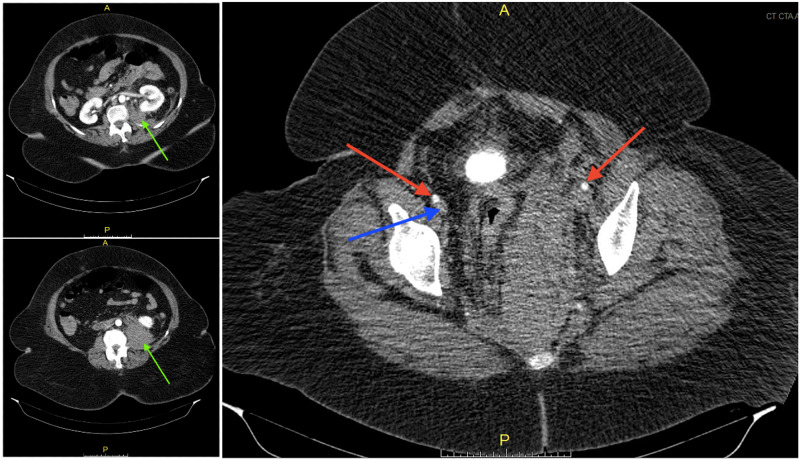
CT angiogram abdominal aorta and bilateral iliofemoral lower extremity runoff with contrast showing a large hematoma (green arrows) extending from the pelvis to the iliopsoas musculature measuring approximately 25 x 10 x 6 cm at the level of the pelvis without extravasation of contrast. The arterial vasculature of the bilateral lower extremities is patent bilaterally (red arrows) but there is greatly impaired venous return at the level of the pelvis with compression of the left common iliac vein by the large hematoma. (Blue arrow is pointing to right femoral vein. Left femoral vein is compressed and not visible.)
